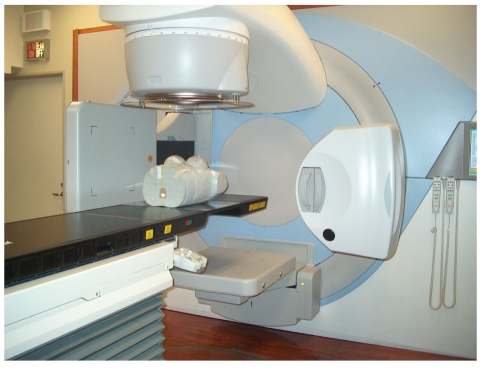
(a) Single exposure port film obtained with Computed Radiography (CR) for 6 MV X-rays; (b) Double exposure port film obtained with Computed Radiography (CR) for 6 MV X-rays.
